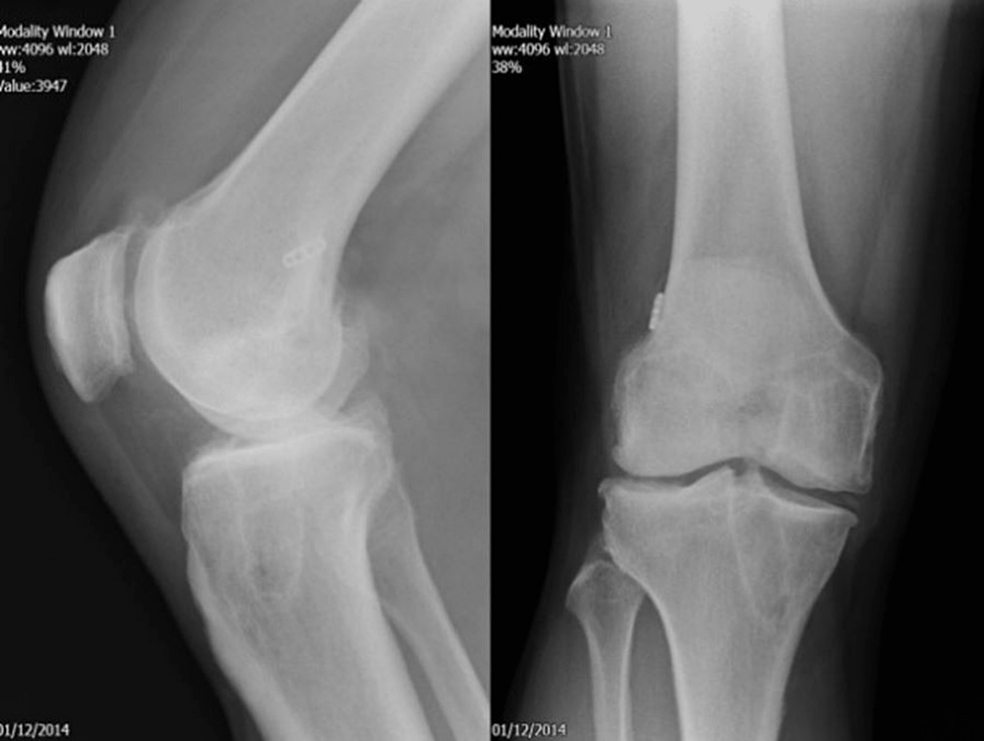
Radiographs of the right knee done in December 2014. Lateral (left) and antero-posterior (right) views showing the tibial and femoral tunnels with a suspensory fixation on the femoral side.
